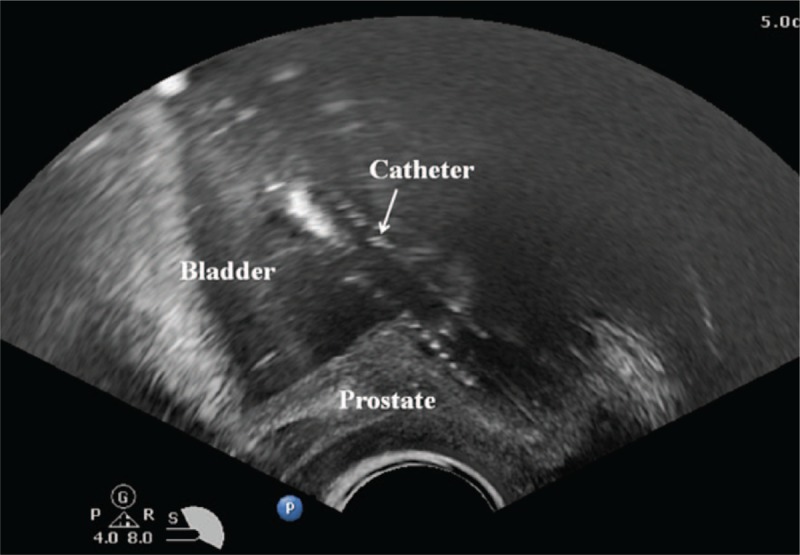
The ultrasonographic findings of urethrovesical junction after successful catheter insertion.
